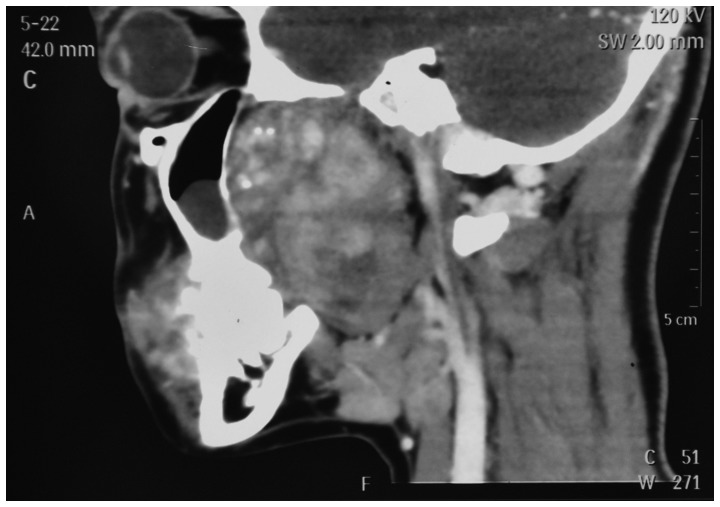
Sagittal computed tomography (CT) scan revealing a soft-tissue mass in the right infratemporal fossa (ITF) and an enlarged foramen ovale.
